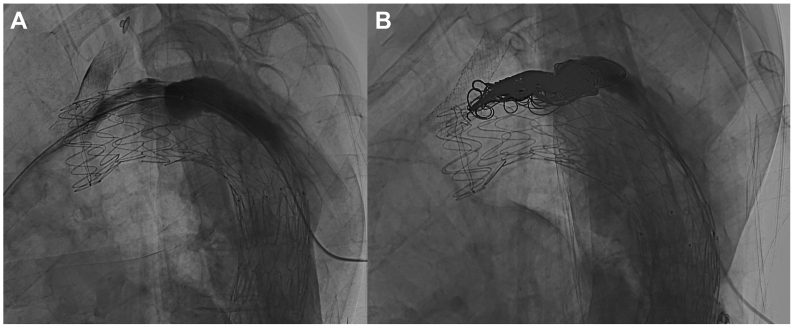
(A) Digital subtraction angiography after transthoracic access to the false lumen showing a type Ia endoleak and (B) subsequent resolution of the type Ia endoleak after coil embolization of the gutter.
